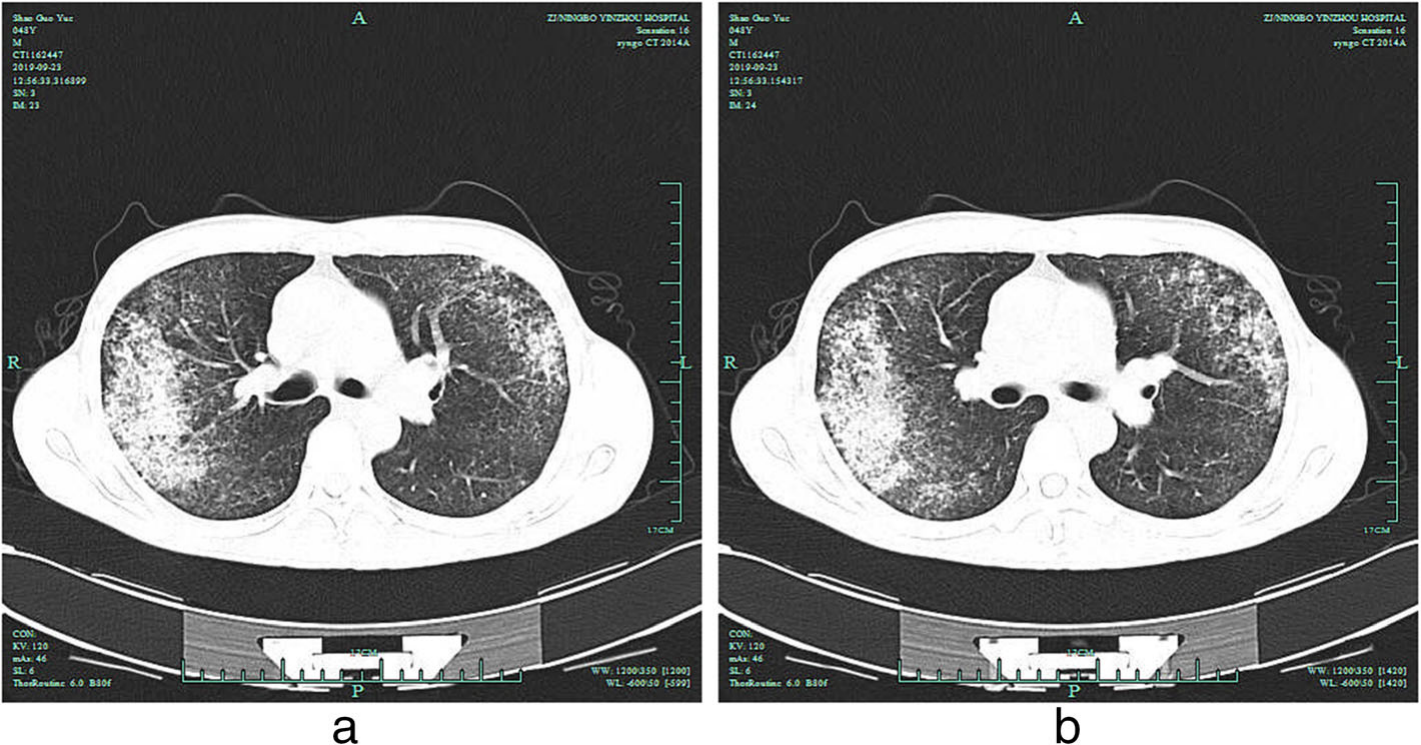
Chest CT on the day of admission. Both lungs presented exudative lesions, which are obvious in the image of the right lung
